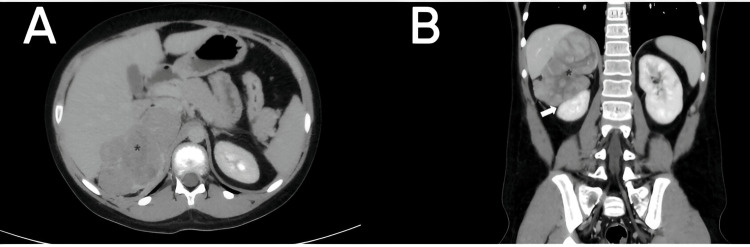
Abdominal computed tomography with intravenous contrast in axial (A) and coronal (B) planes. Mass with heterogeneous enhancement and large size in the right adrenal gland (asterisk in A and B), with loss of cleavage plane with the right lobe of the liver and displacing the kidney downward (arrow in B).
